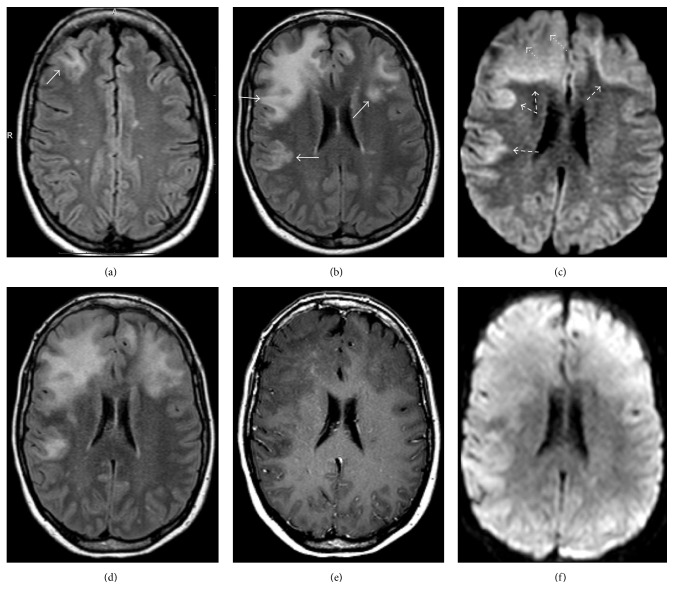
39-year-old woman with RRMS treated with natalizumab developed worsening symptoms. (a) MRI was performed at an outside institution demonstrating a new subcortical lesion in the right frontal lobe (arrow). She was treated for MS exacerbation. The patient presented to our institution approximately 3 months later with progressive symptoms and functional decline. (b) Repeat MRI shows increase in size of the right frontal lesion with characteristic bilateral, asymmetric distribution of lesions involving the subcortical U-fibers (arrows). (c) DWI shows a bright rim of signal along the advancing edge of the lesion (dashed arrows) with darker signal more anteriorly where the lesion is no longer active (dotted arrows). At this time there was no contrast enhancement (not shown). The patient was diagnosed with PML and PLEX was performed. Approximately one month later the patient experienced functional decline. Repeat MRI shows expansion of the (d) FLAIR lesions with increased swelling and mass effect and (e) the development of patchy central enhancement consistent with PML-IRIS. Note that the DWI image (f) no longer demonstrates an advancing edge of restricted diffusion.
